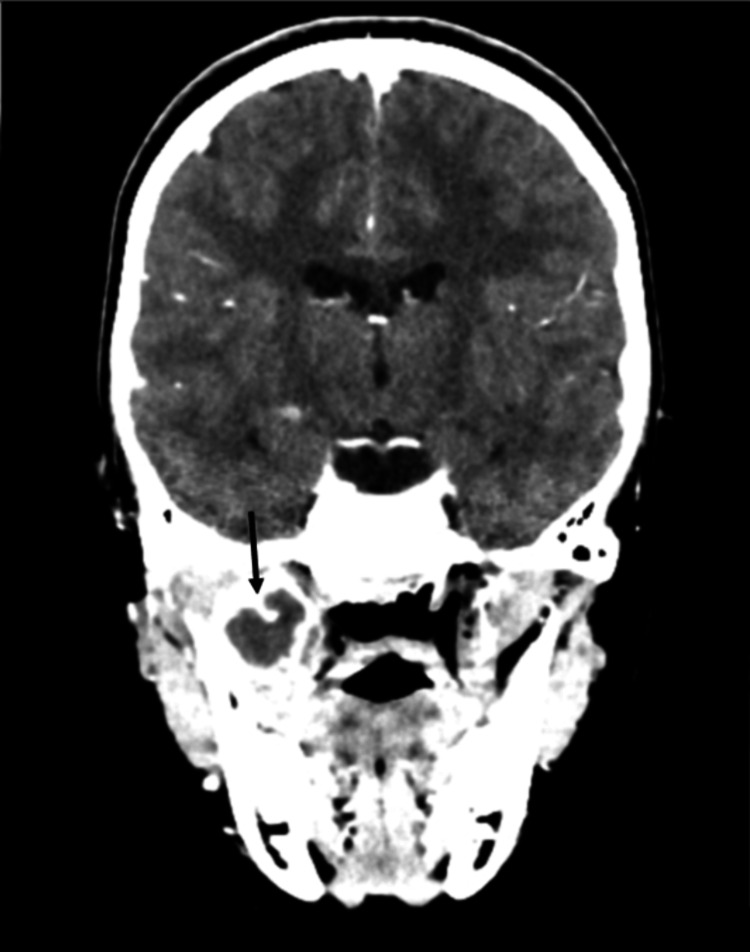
Third hospital admission. Coronal slice CT head with contrast showing a recurrent abscess within the right masticator space
